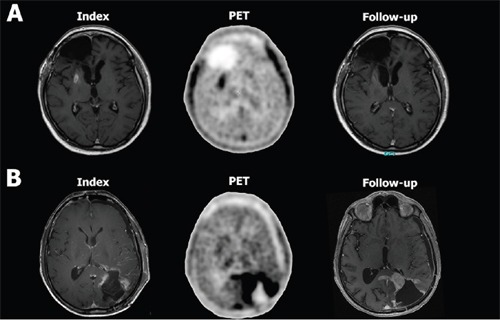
PET image with the corresponding MRI scans from a patient with pseudoprogression (patient 2, A) and true progression (patient 13, B) Figure 1A shows exemplary the index T1 contrast-enhanced (CE) MRI scan where a new CE lesion in the right-sided basal ganglia appeared for the first time. The PET scan next to it shows an increased FET uptake in that area. On follow-up, the CE lesion regressed spontaneously, indicating pseudoprogression. Figure 1B shows exemplary the index T1 CE MRI scan with a new CE lesion around the resection cavity in the posterior lobe. The corresponding PET scan shows extensive FET accumulation far beyond the CE lesion. In the follow-up MRI scan, the index CE lesion increased considerably and thus confirmed true progression. By a mere visual comparison of the PET images from the patient with pseudoprogression A. with that from the patient with true progression B. one can not infer that there is any difference in tracer uptake. Assessing the texture of tracer uptake, however, provides more information than can be delineated by visual inspection.
